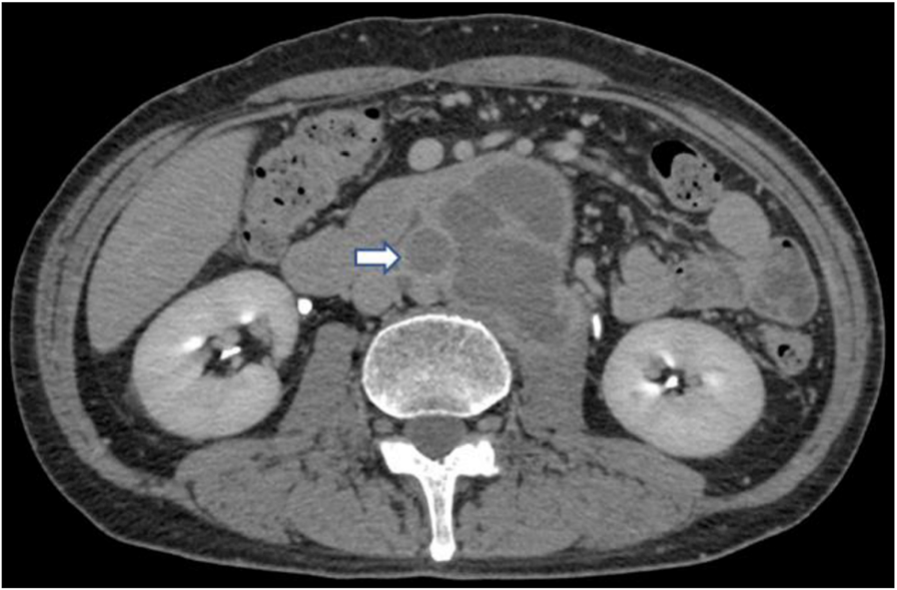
Follow-up enhanced computed tomography at 1 month after the initial examination showing total occlusion of the abdominal aorta (arrow)
